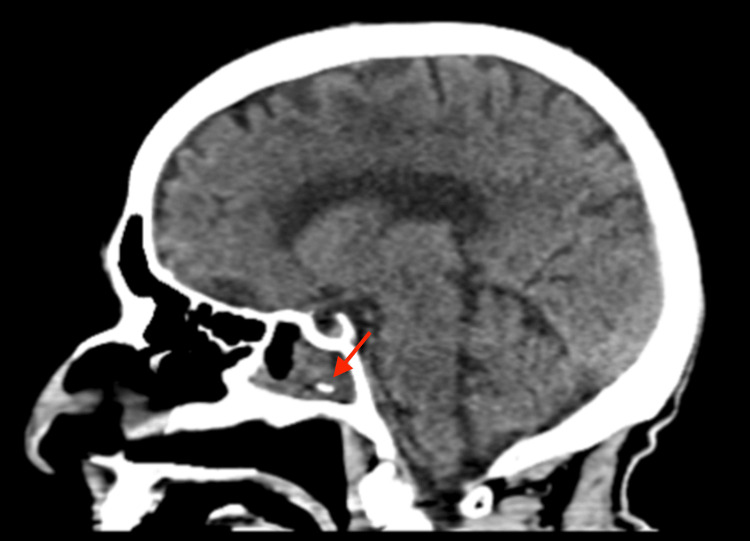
Head CT without contrast (sagittal view) showing calcification in the sphenoid sinus
